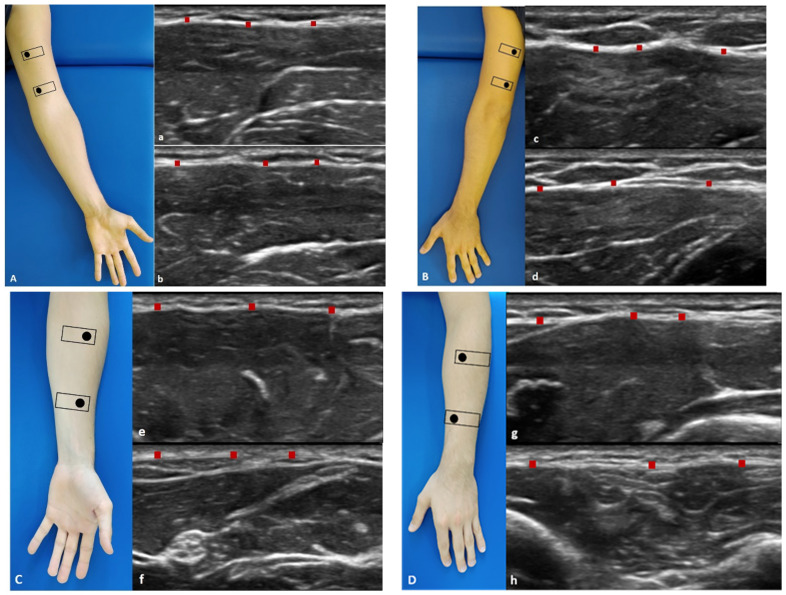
Ultrasound (US) images of the deep fascia of: the anterior region of the arm (A) and of the forearm (C); the posterior region of the arm (B) and of the forearm (D). Anterior region (A,C) at the levels Ant 1 (a,e) and Ant 2 (b,f). Posterior region (B,D) at levels Post 1 (c,g) and Post 2 (d,h). Probe: black rectangle. Red dashes: deep fascia. Reprinted with permission from [14].
